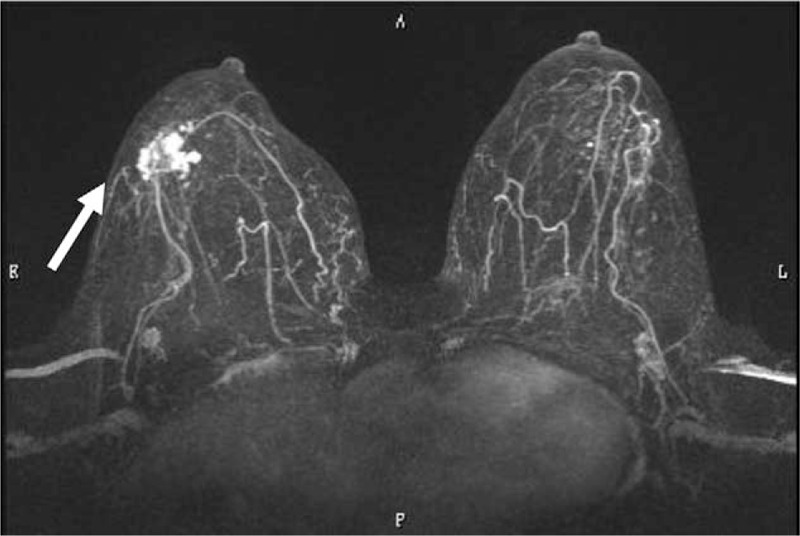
MRI findings. The horizontal view showed a nodular lesion (arrow), which measured 30 × 30 mm. MRI = magnetic resonance imaging.
